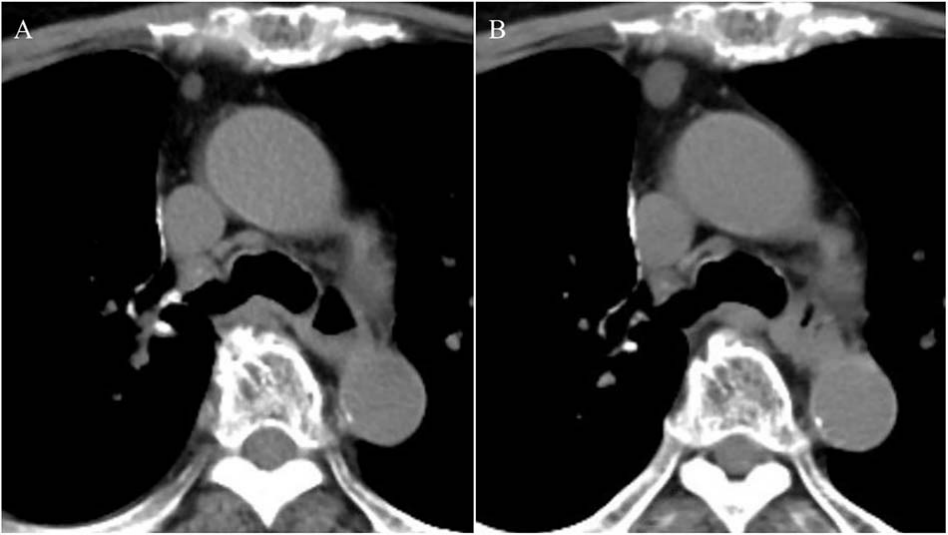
Chest computed tomography showing a small nodule with a diameter of 6 mm at the anterior mediastinum (A); after 2 years, the nodule had enlarged to 13 mm (B).
